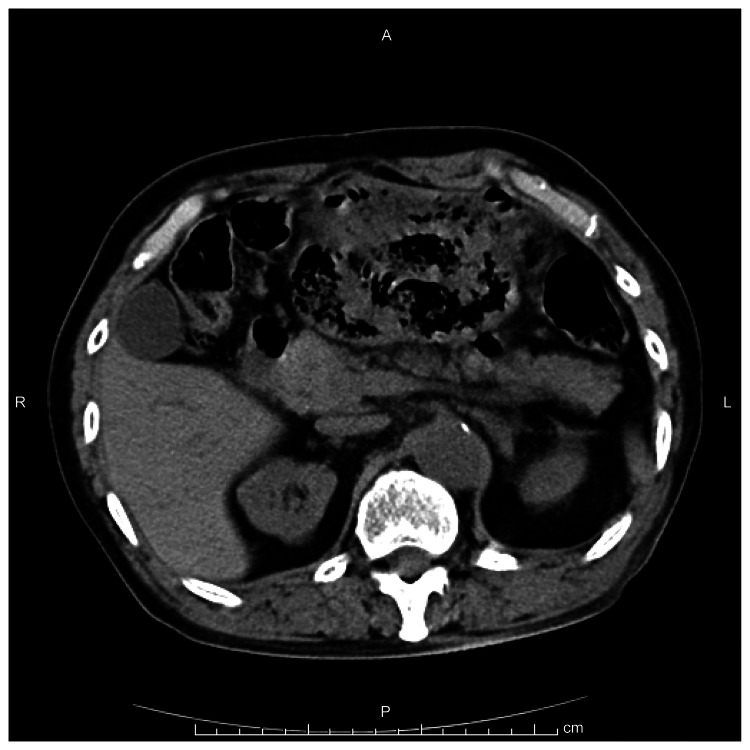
Abdominal computed tomography shows abdominal free air (arrow).
